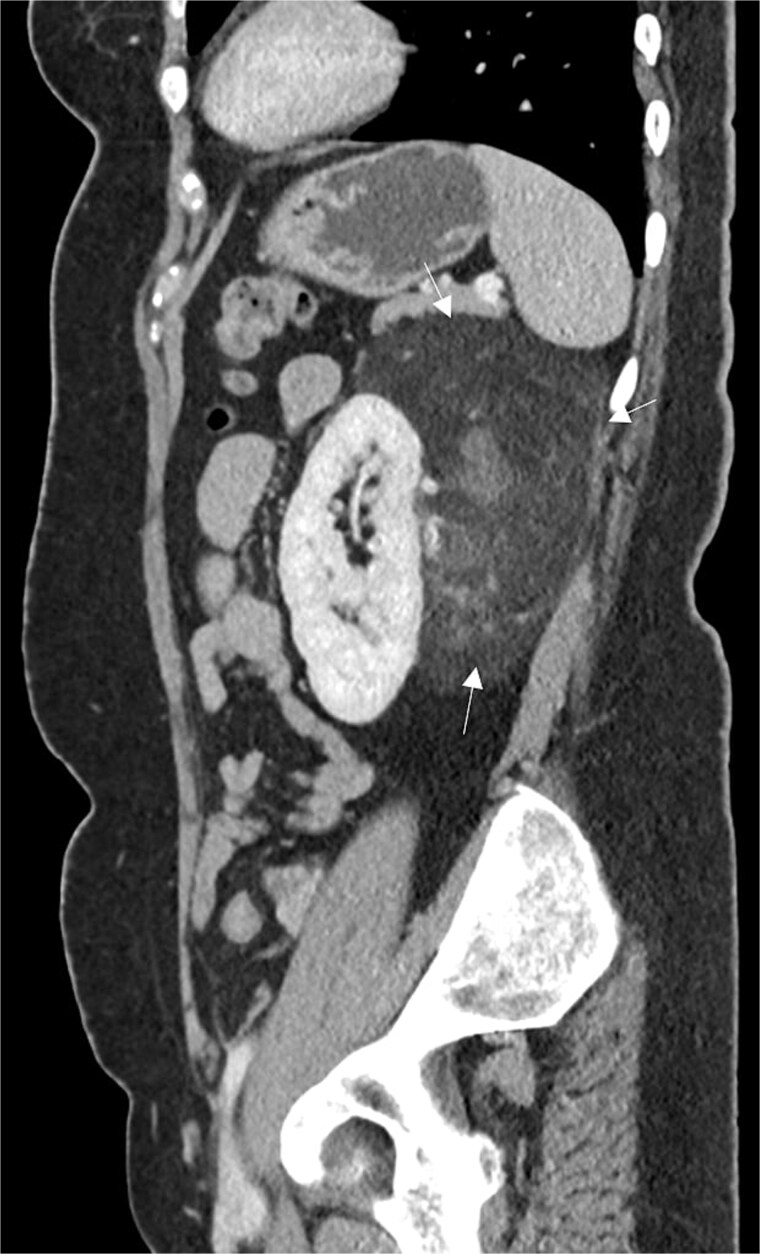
Sagittal CT abdomen and pelvis with contrast depicting a large retroperitoneal mass.
